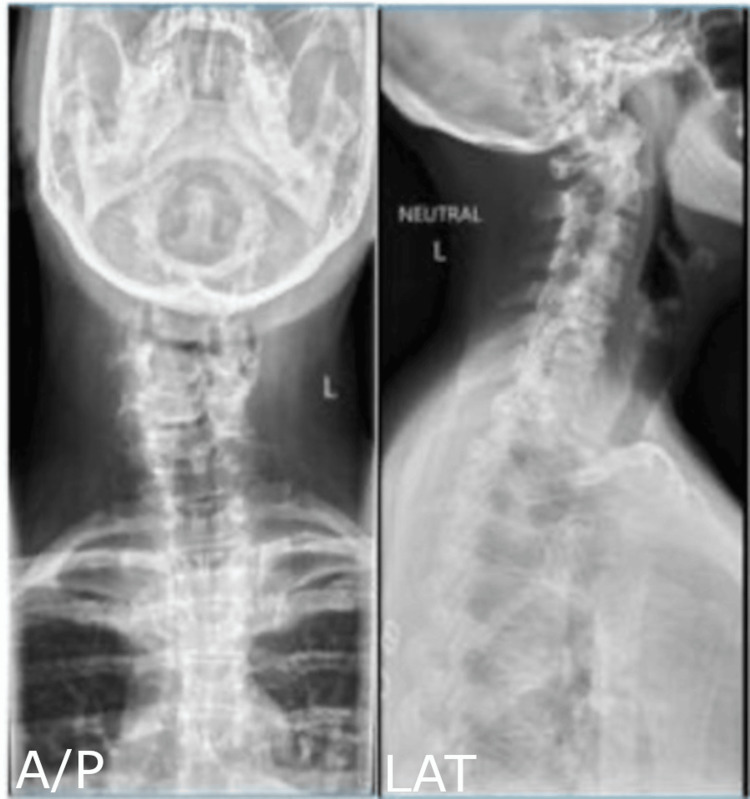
Initial Upright Cervical Radiographs Upright cervical radiographs obtained three years prior demonstrating loss of cervical lordosis, mid-cervical disc degeneration, mild upper cervical scoliosis, and grade I C4-5 spondylolisthesis. A/P: anteroposterior; LAT: lateral
